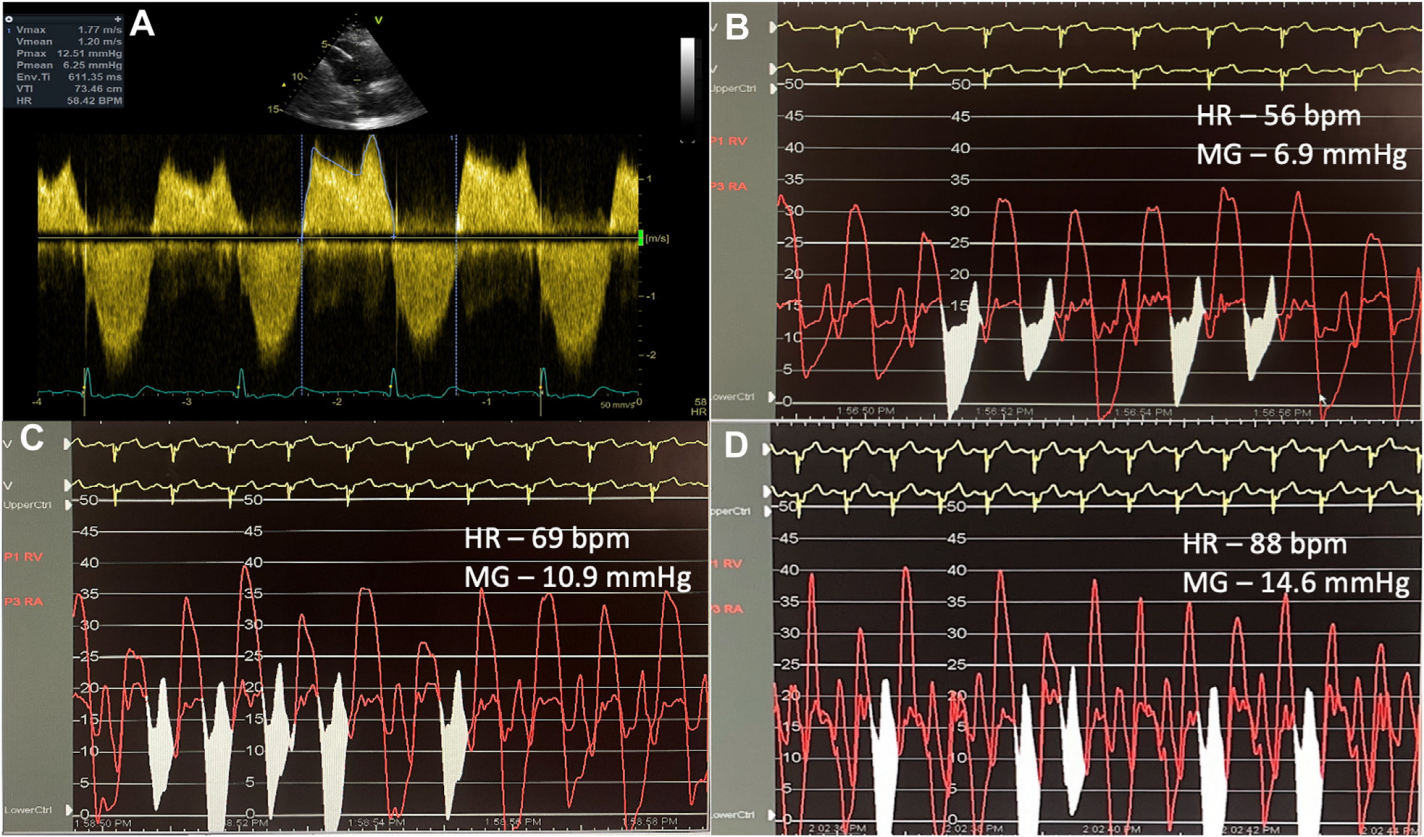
Pressure gradients across the tricuspid valve at rest and with exercise. (A) Doppler signal across tricuspid valve. Pressure gradient measurement across the tricuspid valve at rest (B), with incremental exercise (C), and peak exercise (D). HR, heart rate; MG, mean gradient; VTI, velocity time integral.
